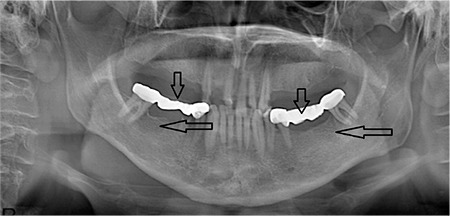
Orthopantogram of the third patient was presented (vertical arrows show the silver amalgam restorations, horizontal arrows show the radiolucent periapical infections in the root of third molar teeth).
